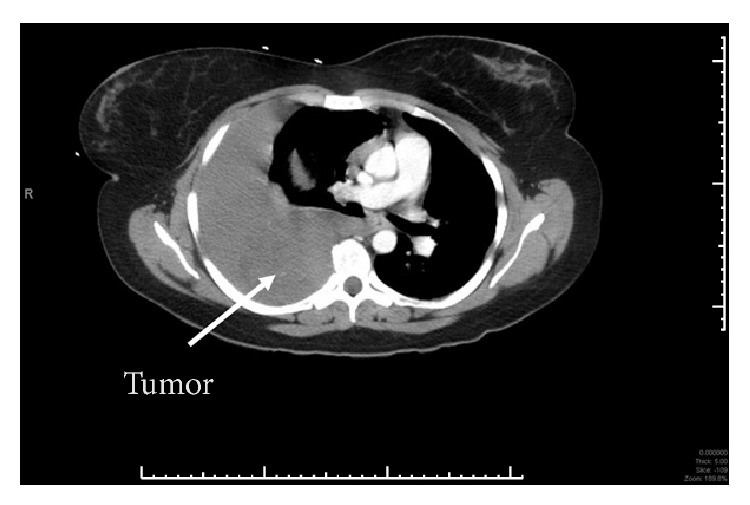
Outside hospital CT scan showing hemothorax and unrecognized schwannoma.
